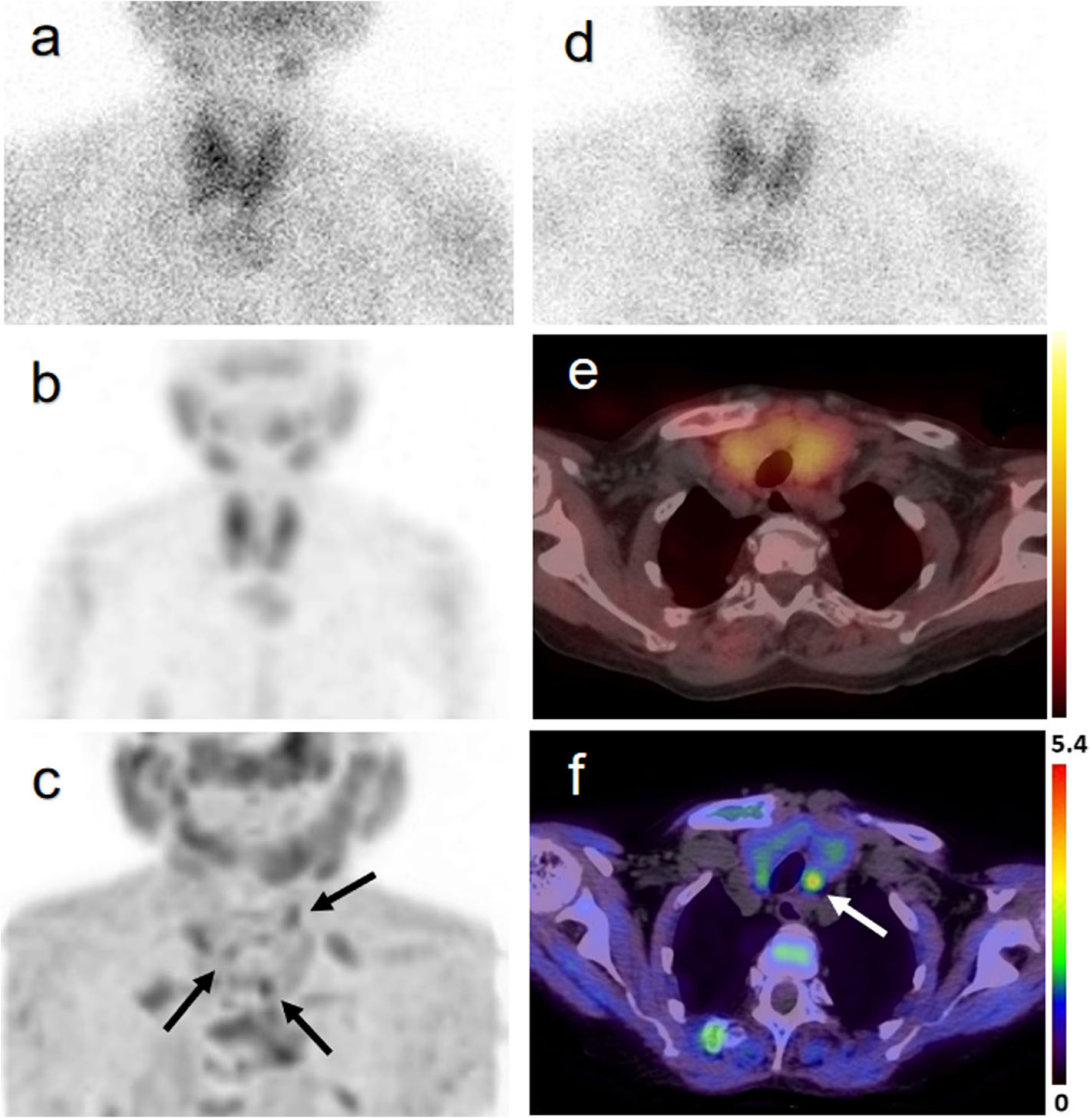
Images of a 62-year-old woman (case 11) with three parathyroid hyperplastic lesions. The early (a) and late (d) planar ^99m^Tc-sestaMIBI SPECT/CT images, MIP (maximum intensity projection) images of ^99m^Tc-sestaMIBI SPECT/CT (b), and fusion image (e) cannot present all three lesions clearly because of Hashimoto’s thyroiditis. Although the MIP image of ^18^F-FCH PET (c) is unclear due to renal osteopathy, the lesion is visible in the image (arrows). An ^18^F-FCH PET/CT fusion image (f) shows homogeneous tracer uptake behind the left lobe of the thyroid gland (arrow)
